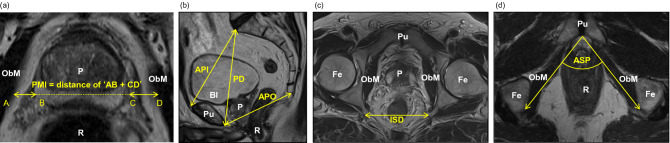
Definition of parameters in MRI measurements. (a) Axial section of MRI on T2 showing the maximum area of the prostate. A dashed-yellow line ‘BC’ indicates the maximum length of the width of the prostate at the section showing the maximum area of the prostate. The line is extended where it crosses the lateral edge of the obturator internus muscle. Point A and D were defined as the point crossing the lateral edge of the obturator internus muscle. ‘Prostate-muscle index (PMI)’ was defined as the sum of distance 'AB + CD' shown in a yellow line with arrowheads. (b) Sagittal section of MRI on T2 showing the pelvic cavity. ‘Anteroposterior diameter of the pelvic inlet (API)’, ‘pelvic depth (PD)’, and ‘anteroposterior diameter of the pelvic outlet (APO)’ were defined as the ‘diameter from the sacral promontory to the most superior aspect of the pubic symphysis’ (mm), ‘diameter from the sacral promontory to the most inferior point of the pubic symphysis’ (mm), ‘diameter from the inferior aspect of the pubic symphysis to the tip of the coccyx’ (mm). (c, d) Axial section of MRI onT2 showing the measurement of ISD and ASP. ‘Interspinous distance (ISD)’ was defined as ‘the narrowest distance between tips of the ischial spines’ (mm). ‘Angle of the symphysis pubis (ASP)’ was also measured. P: prostate, Fe: femoral bone, Pu: pubic bone, R: rectum, ObM: obturator internus muscle, Bl: bladder.
